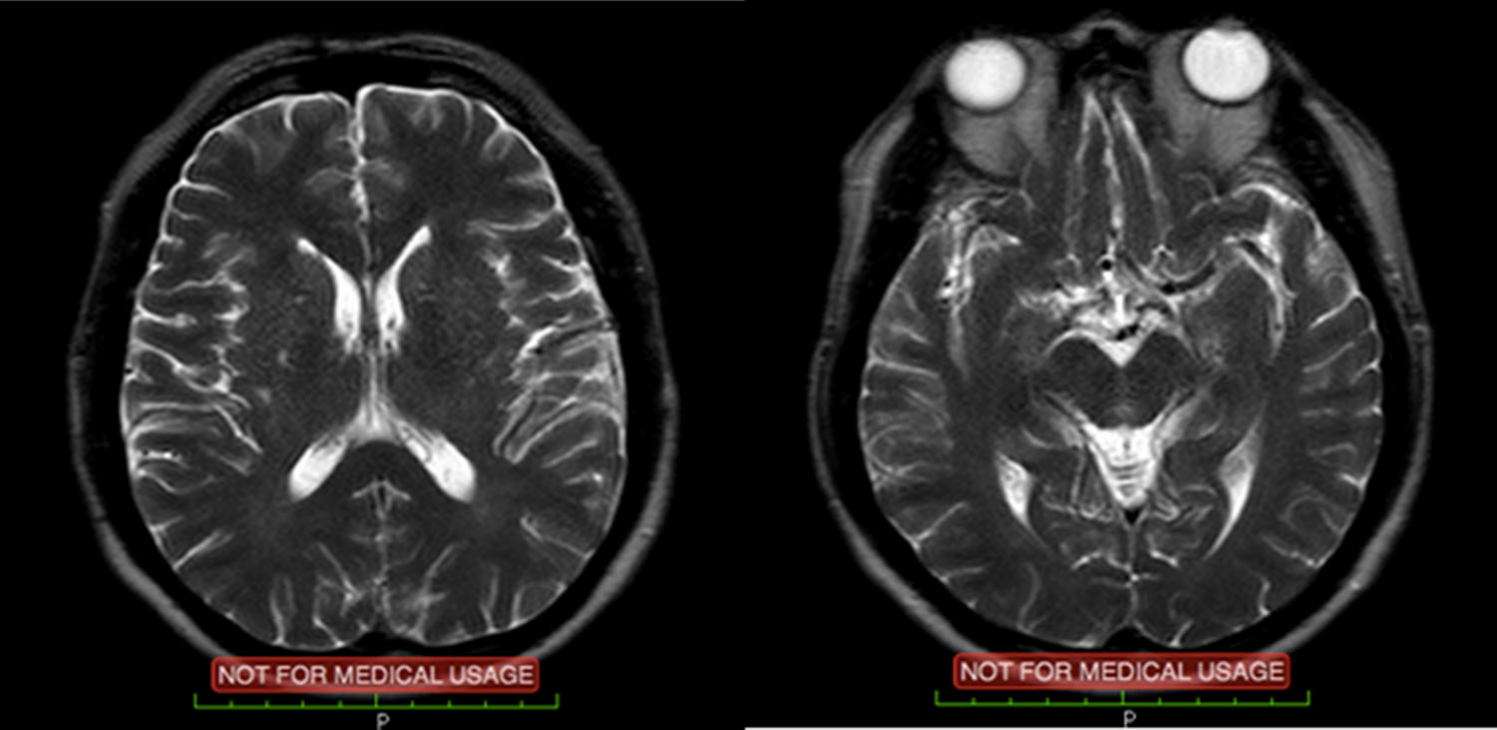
Brain magnetic resonance imaging (MRI) showing no signs of acute infarction or hemorrhagic processes.
